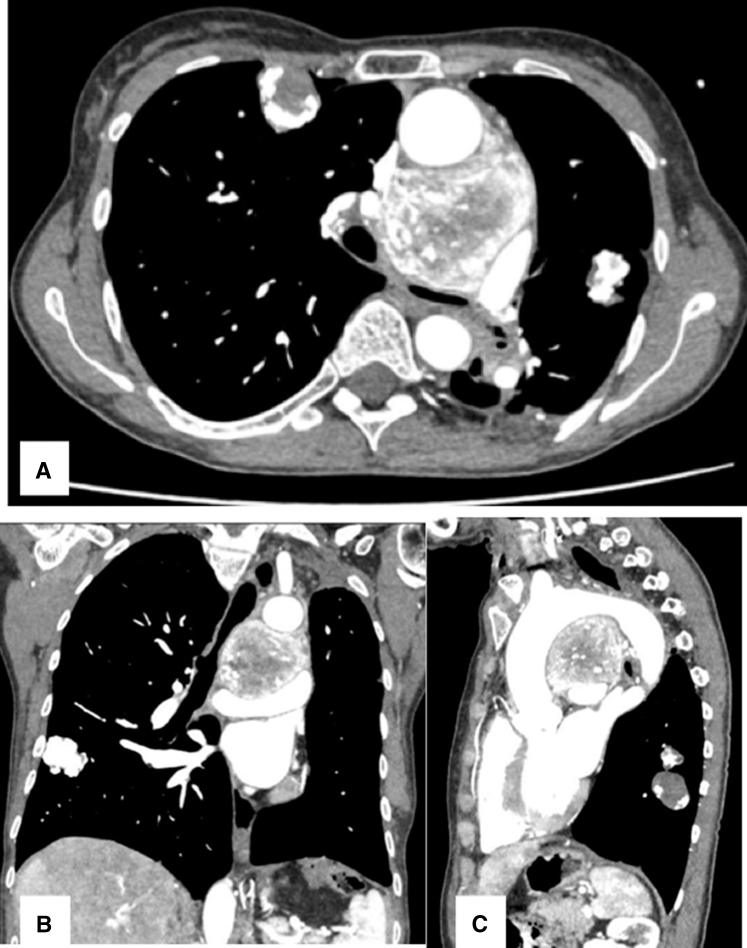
A-C, Contrast-enhanced preoperative computed tomography scan of the chest in sagittal, coronal, and axial views showing a mass of the aortopulmonary window measuring 65 × 60 × 52 mm, located posterior to the ascending aorta, below the aortic arch, above the right pulmonary artery, medial to the superior vena cava, and anterior to the carina and the left main bronchus.
